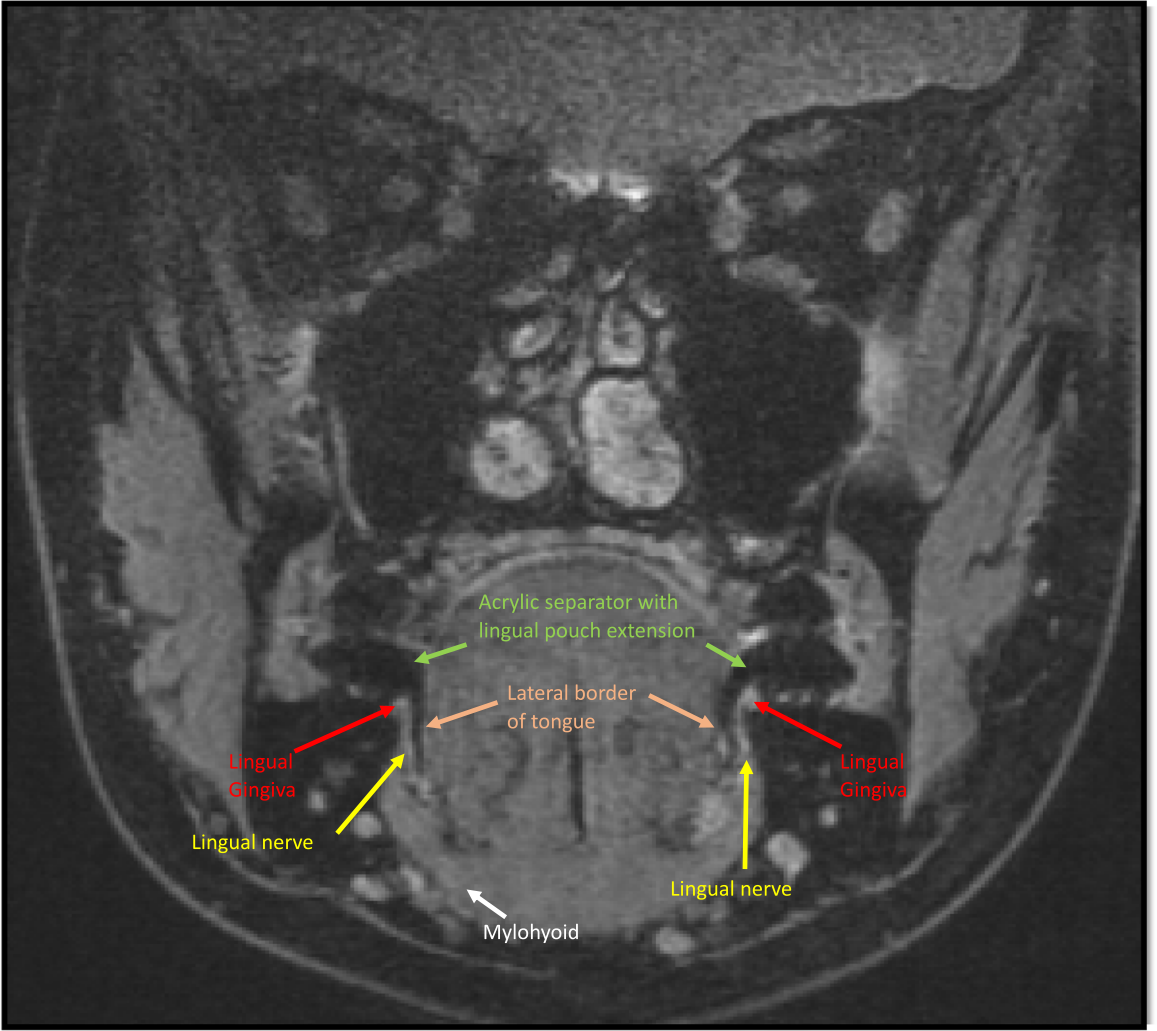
Annotated MRI coronal view of T2 3D-DESS WE at the level of an erupted third molar region, (point B) notice the intra-oral separator in black giving clear demarcation of the tongue and lingual mucosa of the mandible. Structures identified—lingual nerve (yellow arrow), lingual gingiva (red arrow), lateral border of tongue (pink arrow) and mylohyoid muscle (white arrow)
